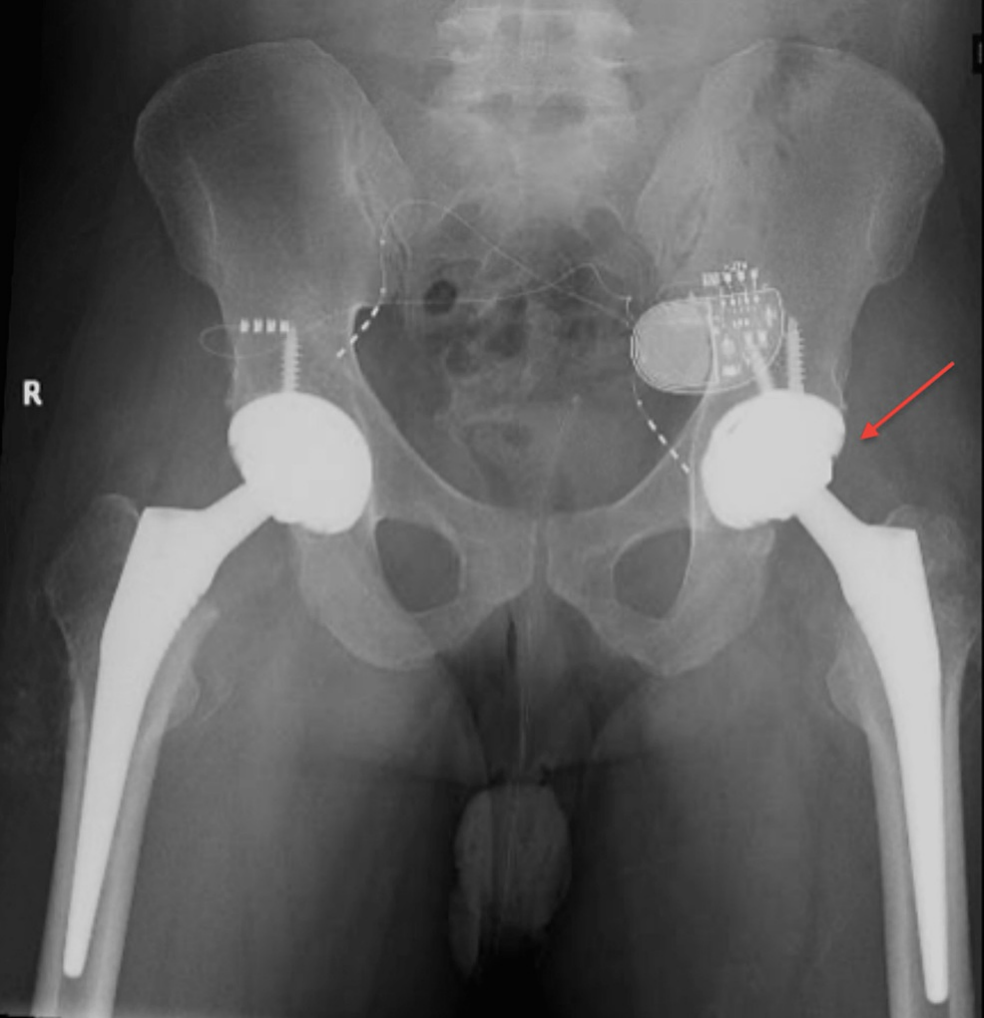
Postoperative left side total hip arthroplasty x-ray
